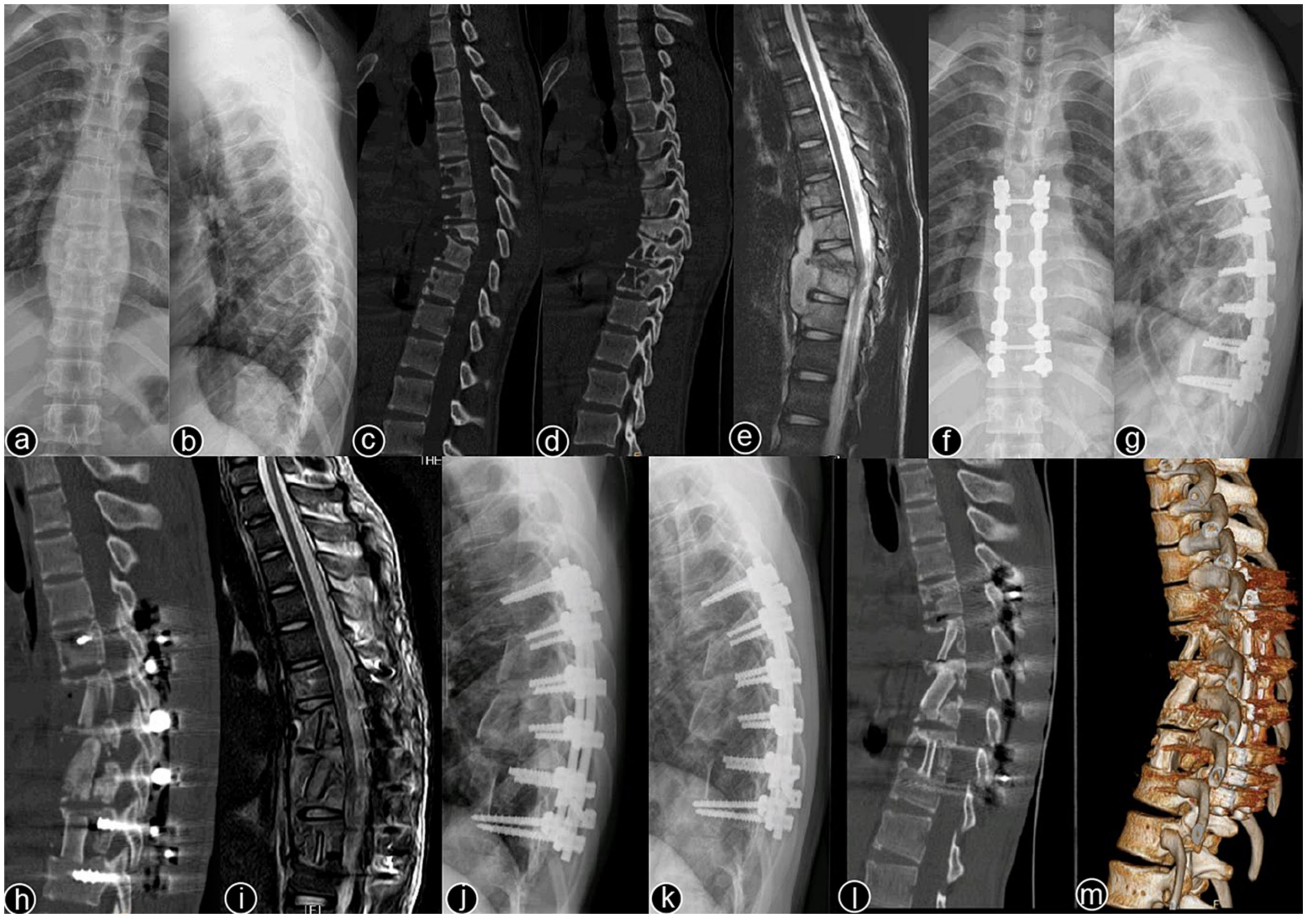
Patient, male, 51 years old. Diagnosis: spinal tuberculosis (T6–T11). Surgical approach: intervertebral surgery. (A,B) Preoperative anteroposterior and lateral X-rays of the thoracic spine show roughened anterior margins of the T6–T11 vertebrae, narrowed intervertebral spaces, and kyphotic deformity. (C,D) Preoperative thoracic spine CT reveals narrowing of the intervertebral spaces, destruction of multiple vertebral bodies, paravertebral soft tissue swelling, and significant vertebral collapse with kyphotic deformity. (E) Preoperative thoracic spine MRI shows multiple abnormal vertebral signals, destruction of vertebral bodies and intervertebral spaces, and the formation of paravertebral abscesses. (F–I) Immediate postoperative anteroposterior and lateral X-rays, CT, and MRI of the thoracic spine show short-screw fixation at T7–T10, complete lesion removal, and satisfactory bone graft placement. (J) One-month postoperative lateral X-ray shows good internal fixation position with effective correction of the kyphotic deformity. (K–M) One-year postoperative lateral X-rays, CT, and 3D reconstruction show no loosening or breakage of the internal fixation, good bone graft positioning, and blurred bone graft interfaces, indicating healing.
